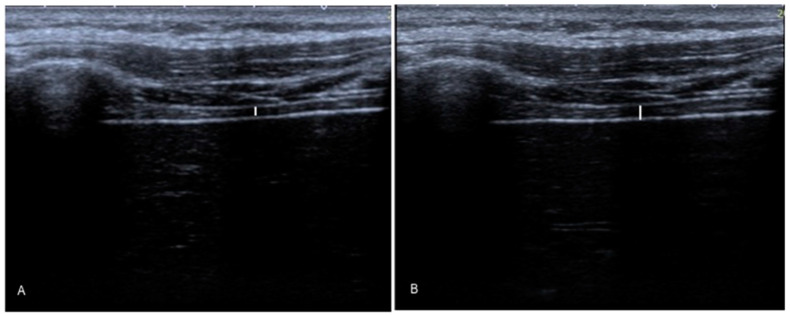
Ultrasound imaging of the hemi-diaphragm by linear probe at the final relaxed expiration (T^exp^; A) and final relaxed inspiration (T^ins^; B).
